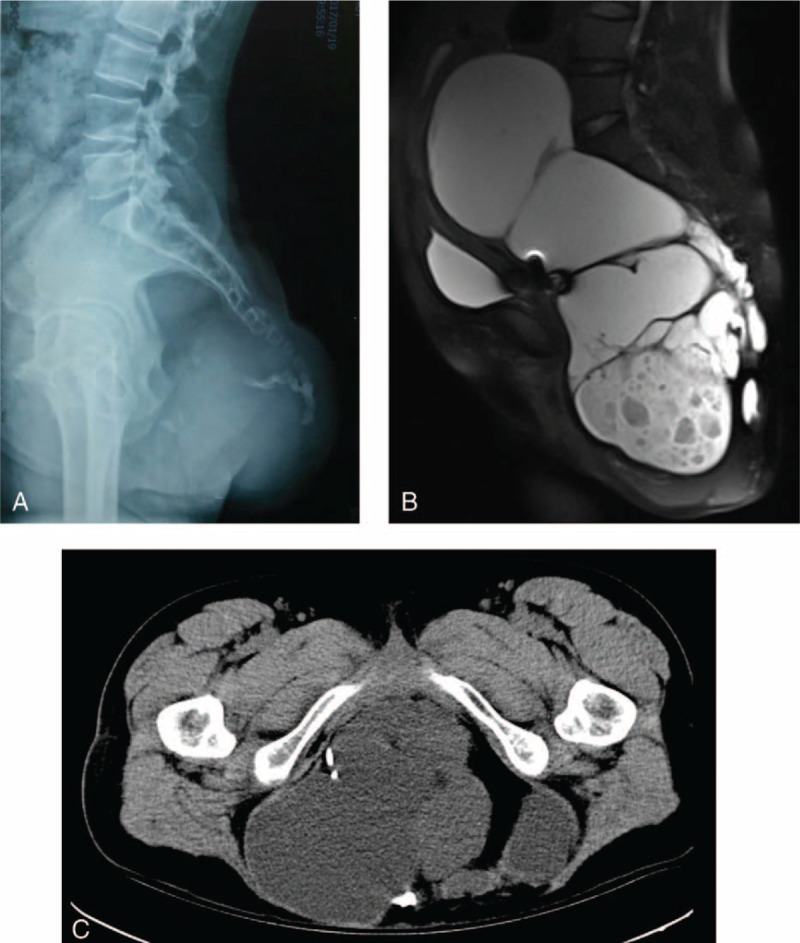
Imaging findings. A large presacral tumor can be seen on plain radiographs, which had invaded the coccyx (A). Enhanced magnetic resonance imaging showed a presacral heterogeneous tumor containing 3 multiloculate cystic areas, one of which presented an enhanced cystic lesion with a solid small nodule component (B). Abdominopelvic computed tomography showed a presacral heterogeneous tumor, 15.6 cm × 12.2 cm × 30.0 cm in size, containing numerous thin-walled and solid components with calcification and fat (C).
